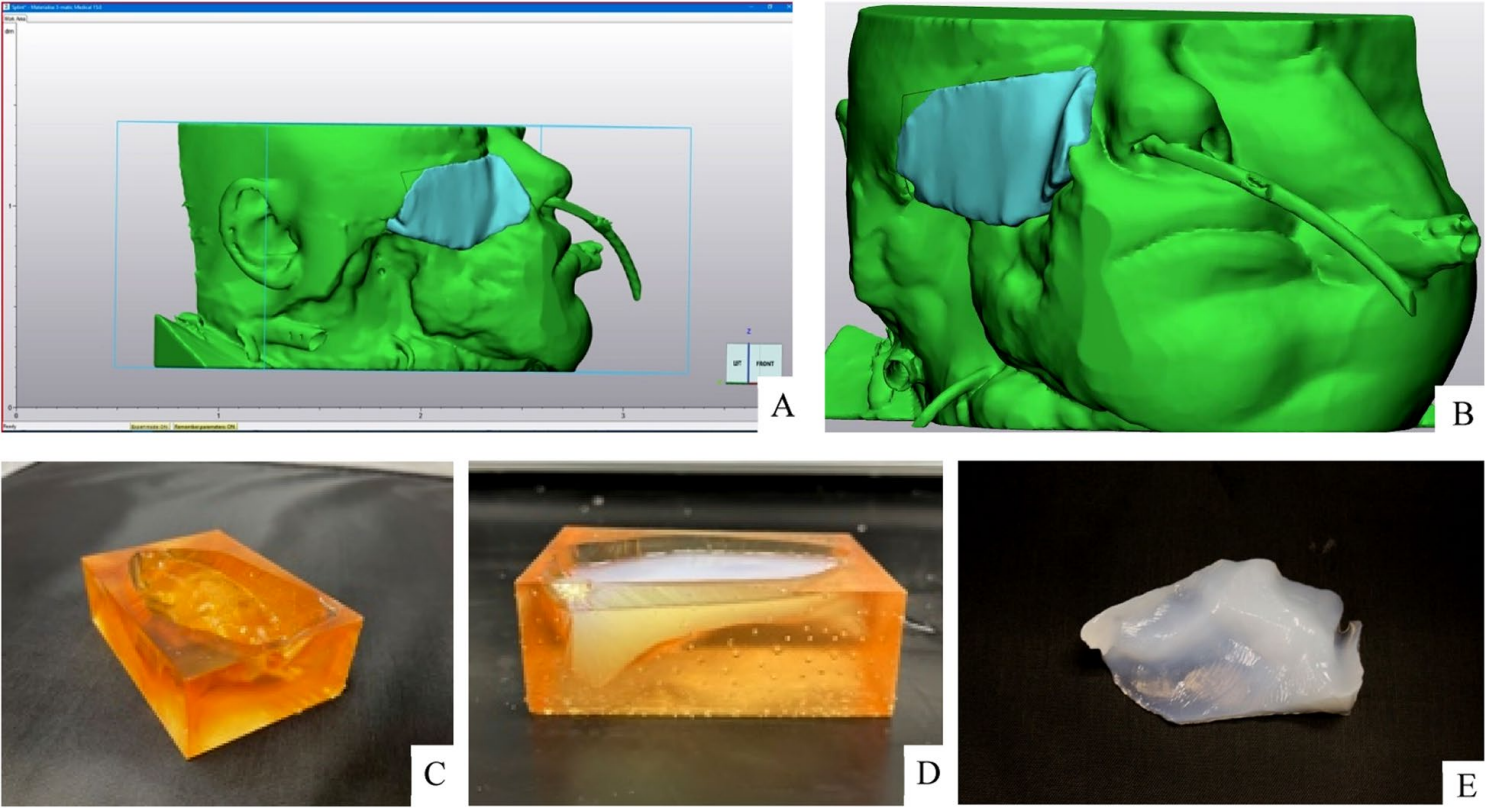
a, b) Design of wound splint based on patient computed tomography (CT) scan c) 3-dimensionally printed device mold d) Pouring of medical grade silicone into mold e) custom wound splint
